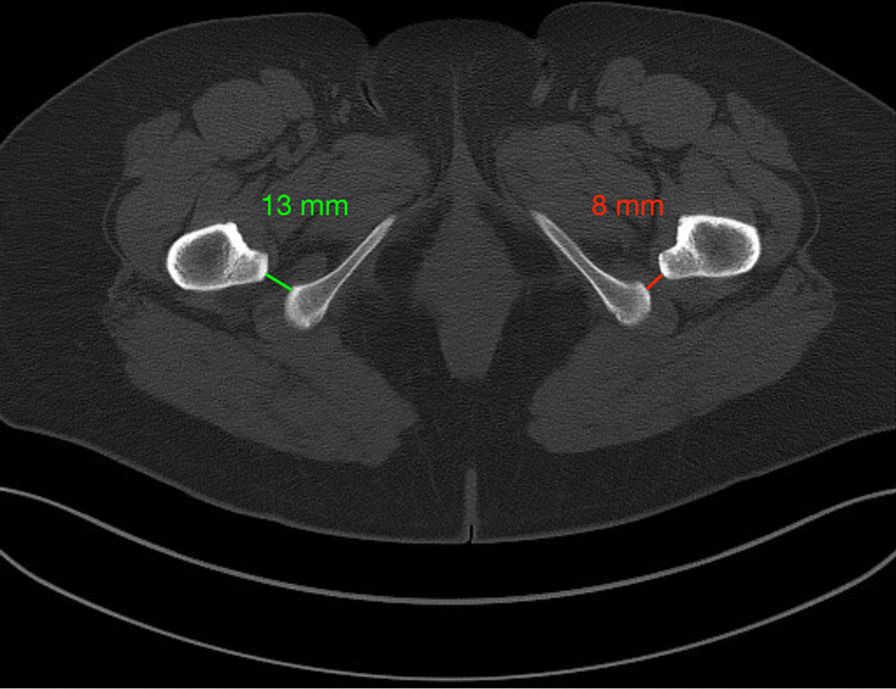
Axial computed tomography slide at level of lesser trochanters. The ischiofemoral distance is smaller on the left than right side
